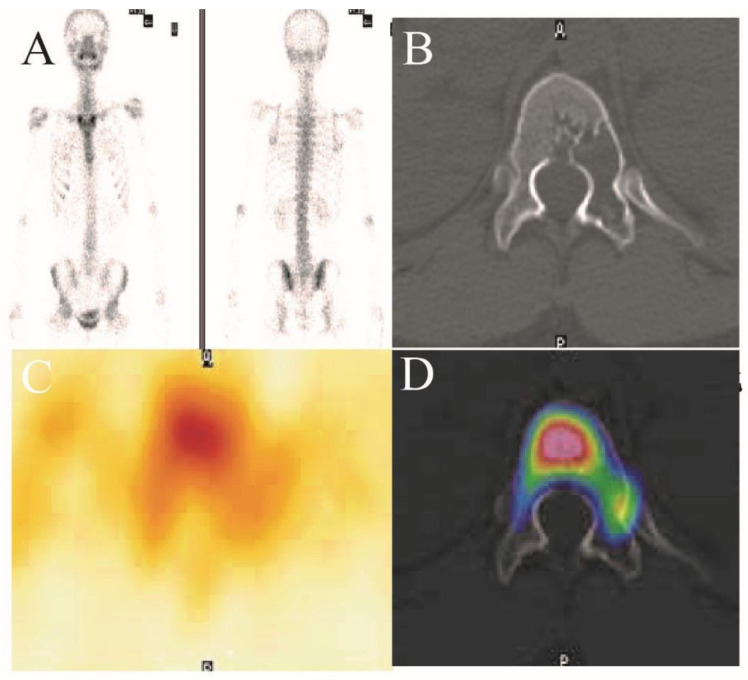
(A–D). Whole-body bone imaging, CT image, SPECT image, and SPECT/CT fusion image suggesting that the left posterior portion of the T12 vertebral body and the left accessory bone were destroyed, with clear borders and sclerotic margins, without visualizer concentration.
